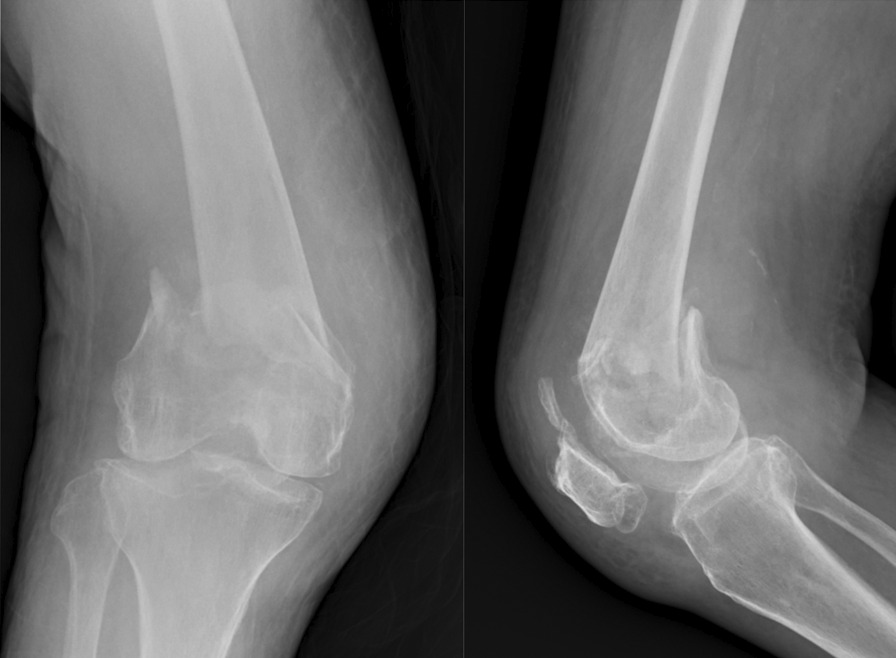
Preoperative radiograph showed distal femur intercondylar fracture
